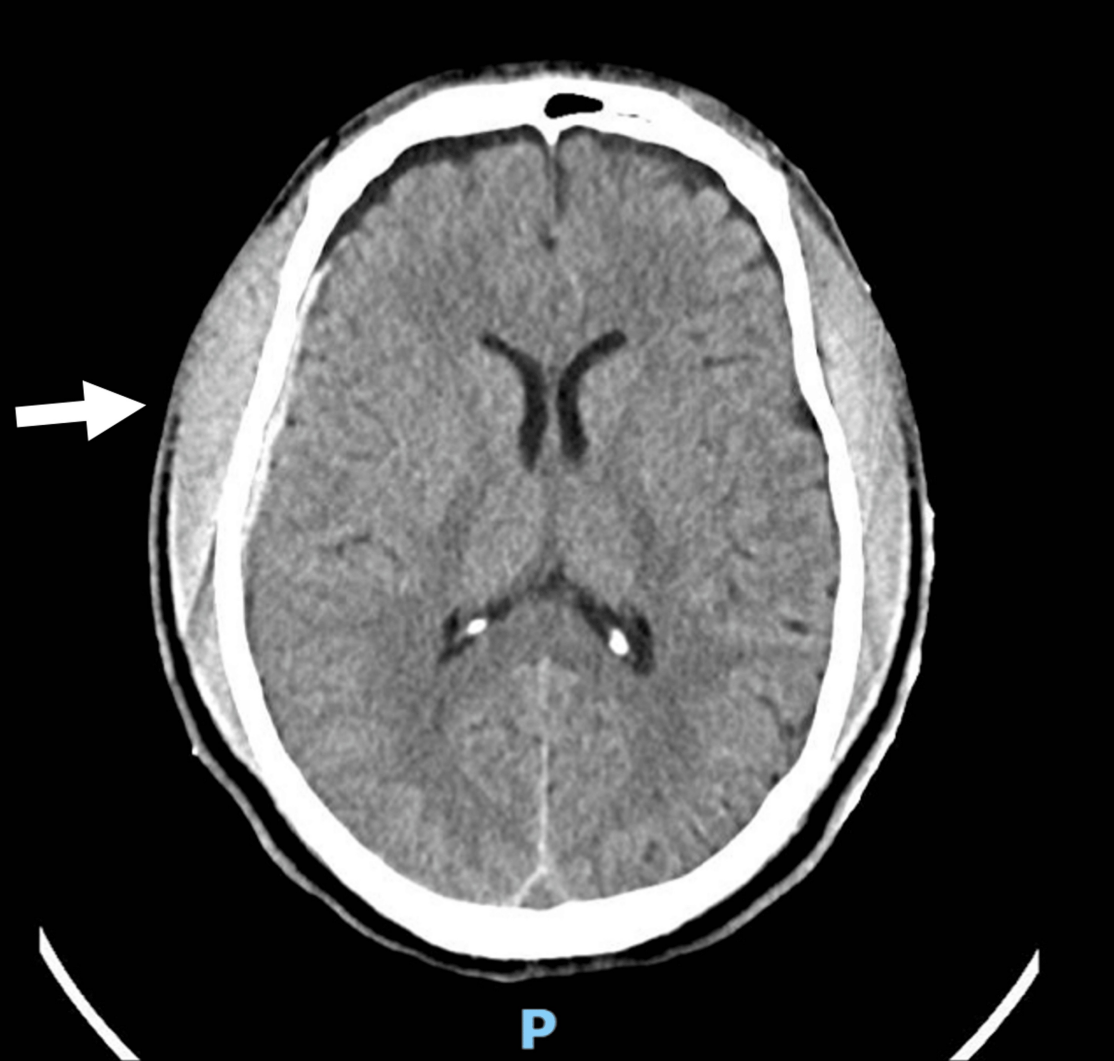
Non-contrast CT brain at presentation demonstrating right frontotemporal subdural haematoma (arrow)
